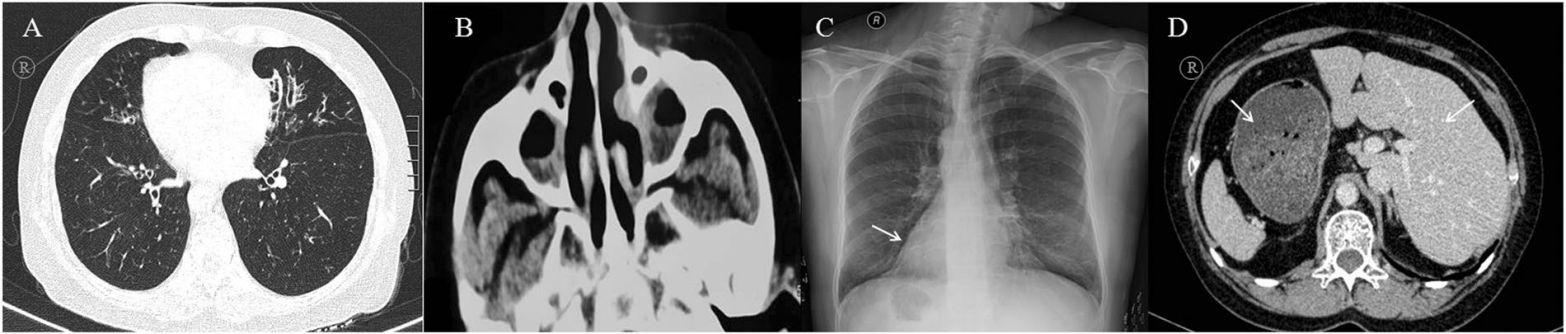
Clinical features of PCD in the consanguineous families. The patient (P6 from family 4) presented the classical clinical features of PCD. (A) The chest computed tomography (CT) scan showed bronchiectasis. (B) The paranasal sinus CT scan of the patient showed apparent rhinosinusitis. Typical situs inversus is shown in C, D. CT images of the remaining patients are shown in Supplementary Figure 1.
